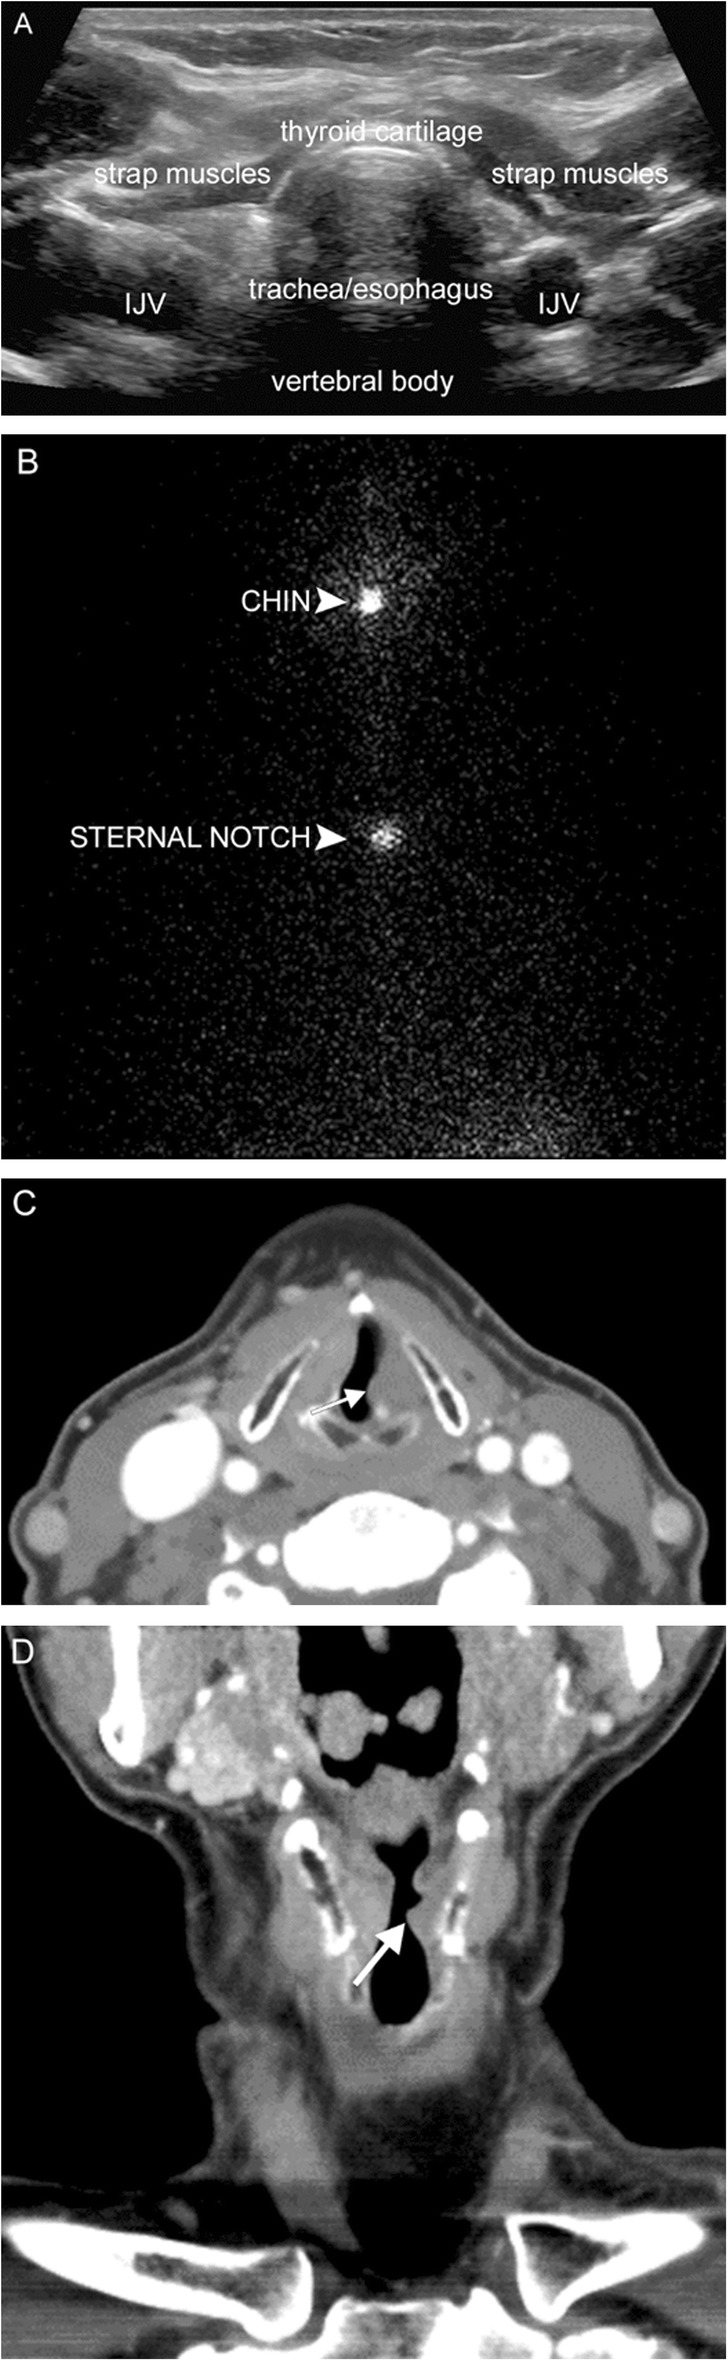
Thyroidectomy. A 63-year-old woman status post total thyroidectomy and radioablation for papillary thyroid carcinoma. Neck ultrasound (a), I-131 whole body scintigraphy (b), and axial/coronal post-contrast CT images (c, d) demonstrate no evidence for residual thyroid tissue in the thyroid bed. In addition, the left vocal fold is medialized (arrow) with dilatation of the left laryngeal ventricle, suggesting vocal cord paralysis due to iatrogenic injury
